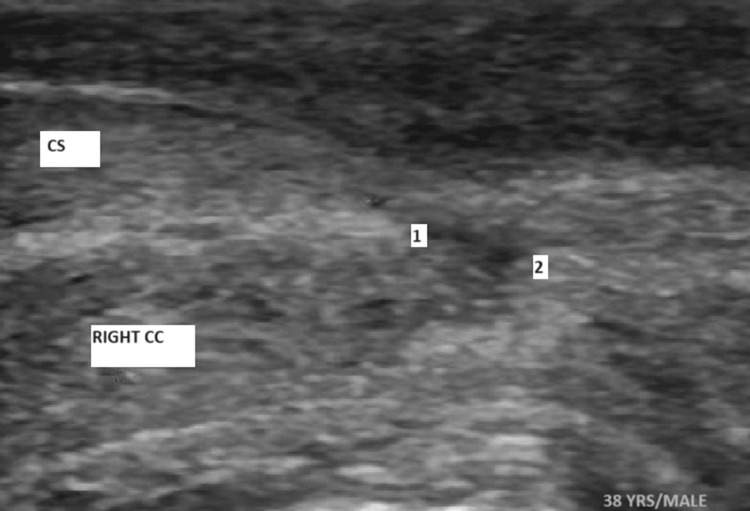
Ultrasonographic image showing a defect in the tunica albuginea (between 1 and 2) of right CC Points 1 and 2 represent the defect in the tunica albuginea. CC: Corpus cavernosum, CS: Corpus spongiosum
